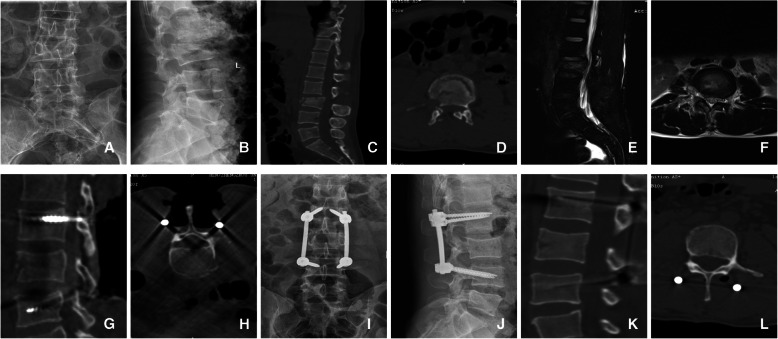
A 23-year-old male presented with a burst fracture of the L 3 vertebral body. Severe back pain with limited mobility and left lower limb muscle strength was grade II. a–f The preoperative imaging data, g, h the intraoperative CT scanning data, and i–l the 12-month postoperative imaging data. Preoperative CT scan showed the fracture fragment burst into the spinal canal (c, d), and MRI showed the dural sac was compressed (e, f).With the assistance of the intraoperative CT, a limited decompression of unilateral lamina was performed, the diameter of spinal canal returned to normal and the pressure of dural sac disappeared (g, h), the result was satisfactory (i-l) 12 months after the operation, and the muscle strength of left lower limb was grade V
